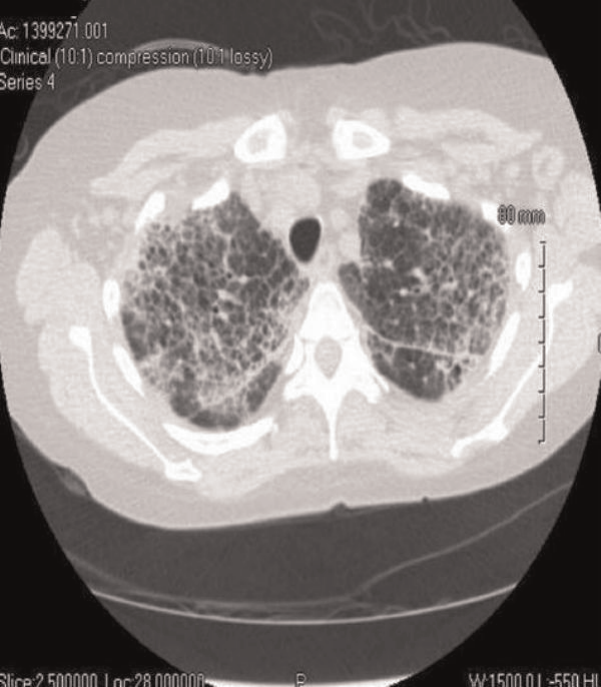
Chest CT revealing diffuse interstitial lung disease with septal interstitial thickening.
